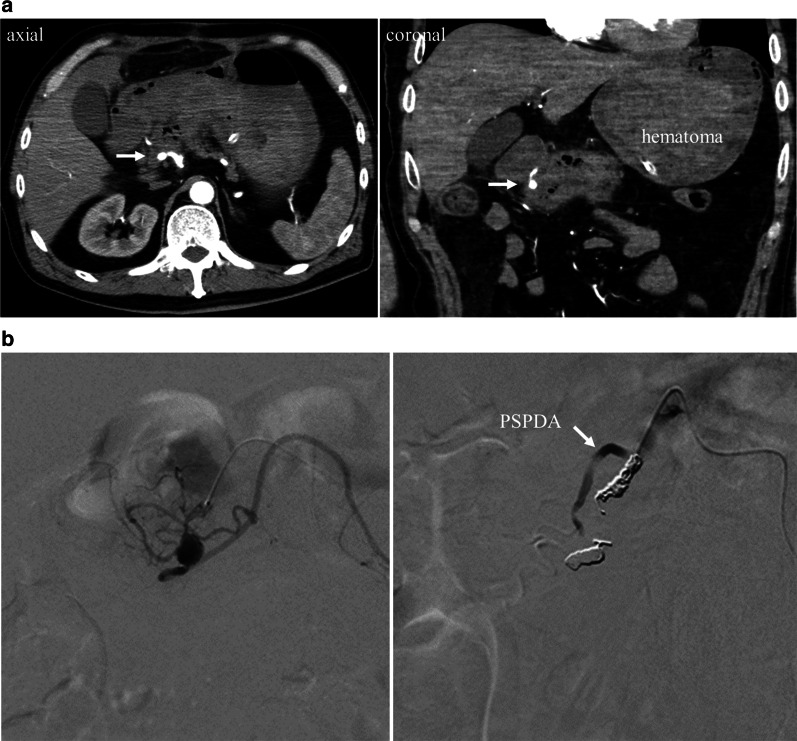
Rupture of the pseudoaneurysm after surgery. a Contrast-enhanced computed tomography revealed media pooled in the duodenal wall as a round 3-mm collection (arrow). b Angiography revealed impending rupture of the pseudoaneurysm causing gastrointestinal bleeding; therefore, the patient underwent coil embolization with preservation of the PSPDA (arrow). *PSPDA* posterior superior pancreaticoduodenal artery
